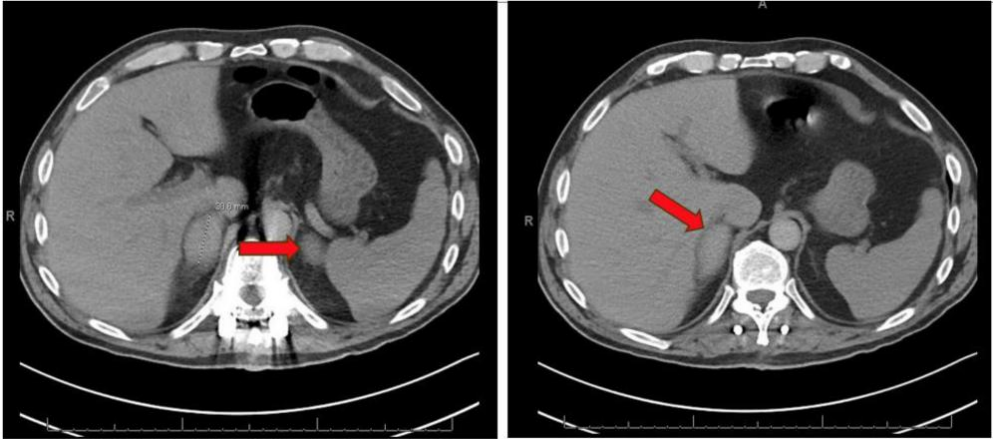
CT angiogram of the chest (pulmonary embolism protocol) revealing hyper-enhancing bilateral enlargement of the adrenal glands post-procedure before the diagnosis of primary adrenal insufficiency.
